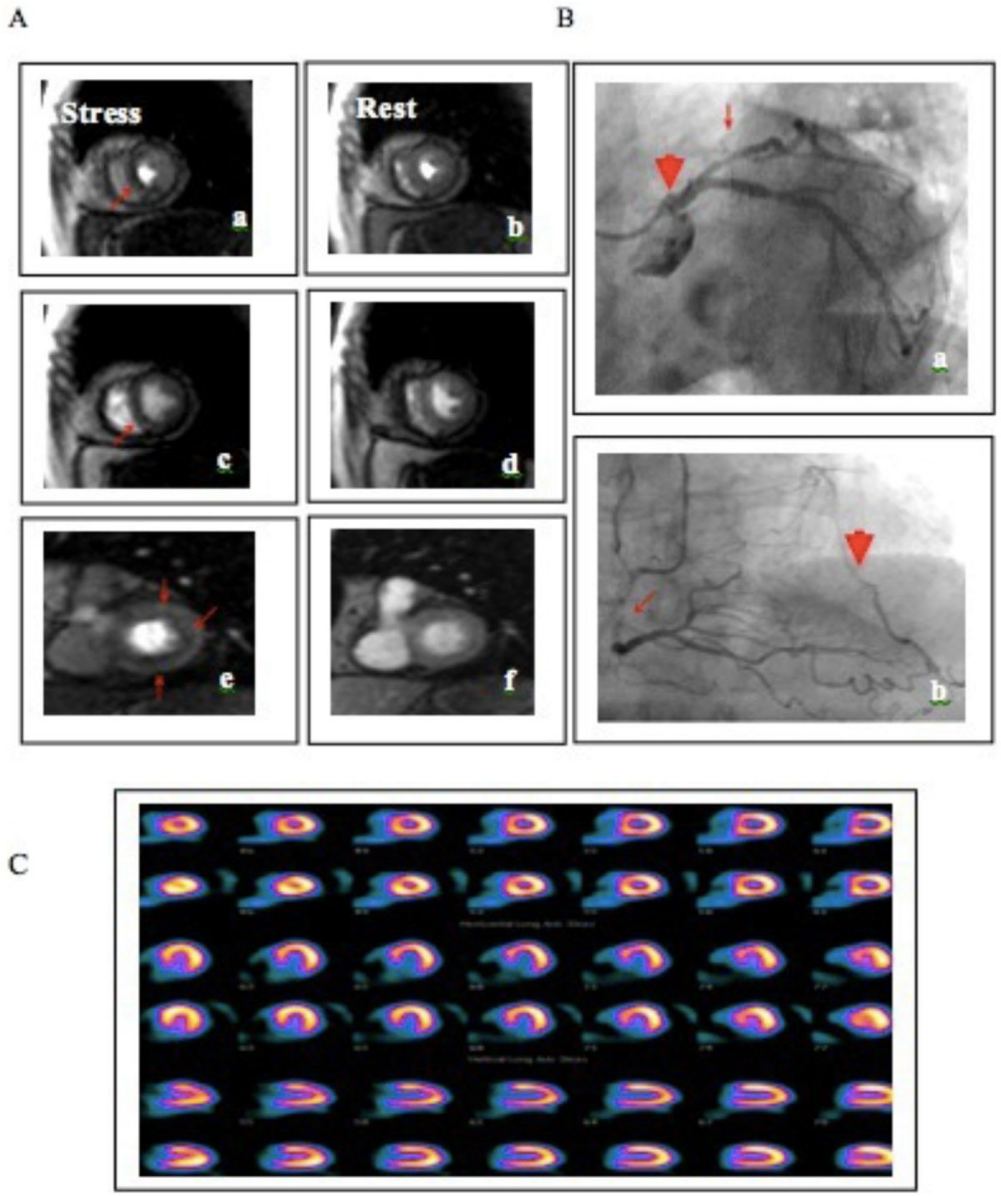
An example of a patient complain of chest pain during stress nearly five years, aggravation a month recently. A. Stress/rest perfusion CMR shows reversible subendocardial hypoperfusion (ischemia) at Apical septal (a), Mid inferoseptal (c), inferior wall (a, c, e), basal anterior and anterolateral wall. B CAG confirmed significant stenosis in all three coronary arteries. The occlusion of the proximal of LAB (B, a arrowhead), RCA collateral blood supply to the distal of LAD (B, b arrowhead) a severe stenosis in the LCX of 80% (B, a arrow). The middle and distal of RCA shows diffuse stenosis of 60-80% (B, b arrow). C. Stress/rest perfusion SPECT shows no significant perfusion defect. As per study protocol, SPECT in this patient was classified as a false negative, showing the potential limitations of SPECT can miss small subendocardial ischemia, and the shortcomings of detecting three balanced coronary lesions.
